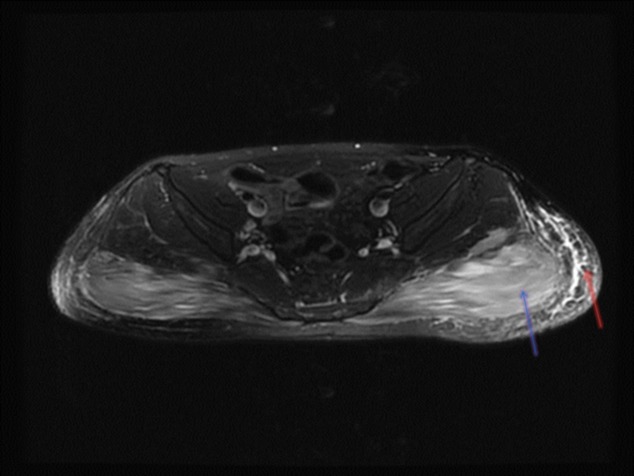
Magnetic resonance imaging of the pelvis. Signs of inflammation of the gluteus maximus muscles bilaterally, more so on the left (blue arrow), and the surrounding fascia (red arrow) are indicated.
